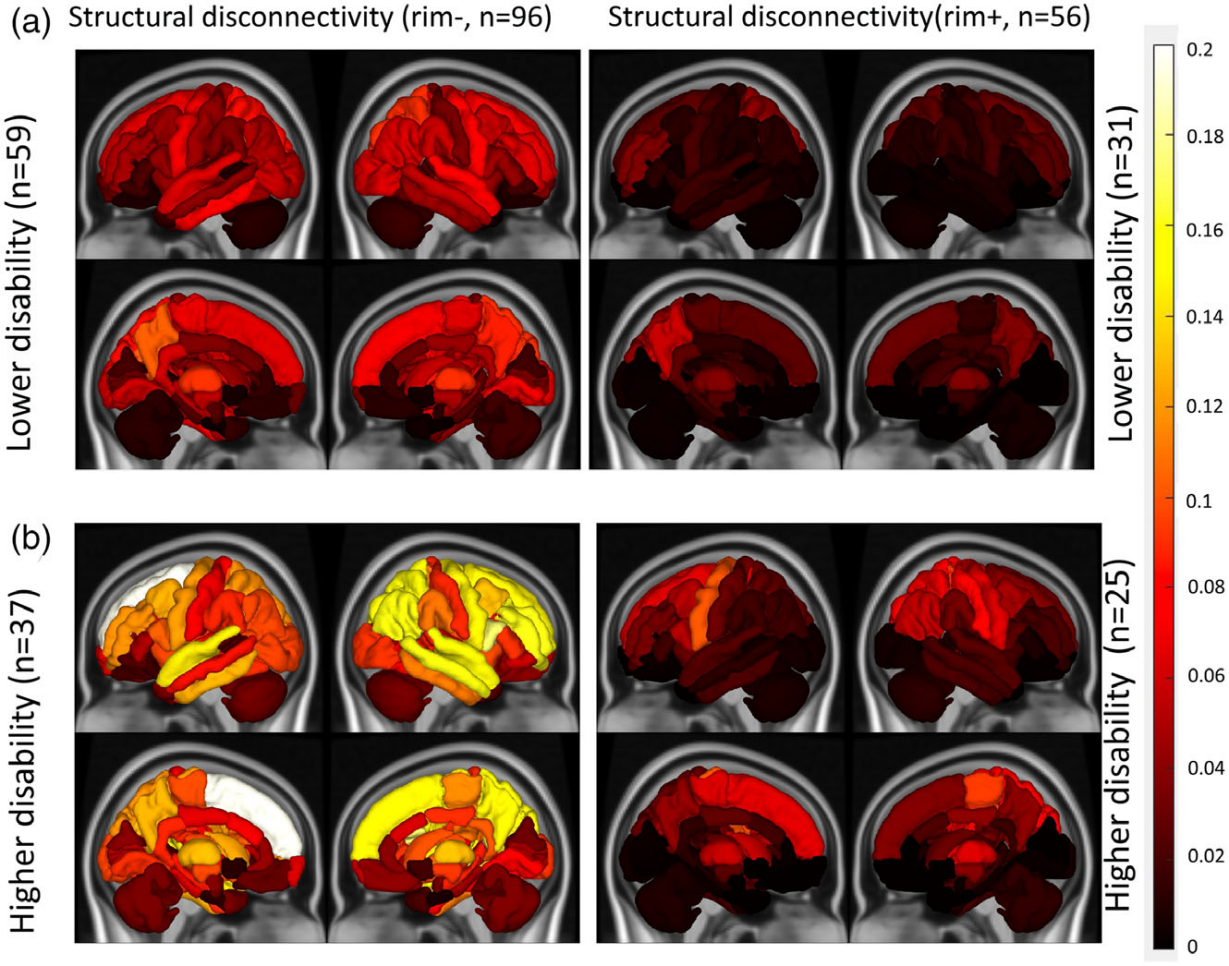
Median of ChaCo extracted from rim‐ (T2 FLAIR lesions excluding rim+ lesions) and rim+ lesion masks for pwMS (A) lower disability versus (B) those with greater disability
